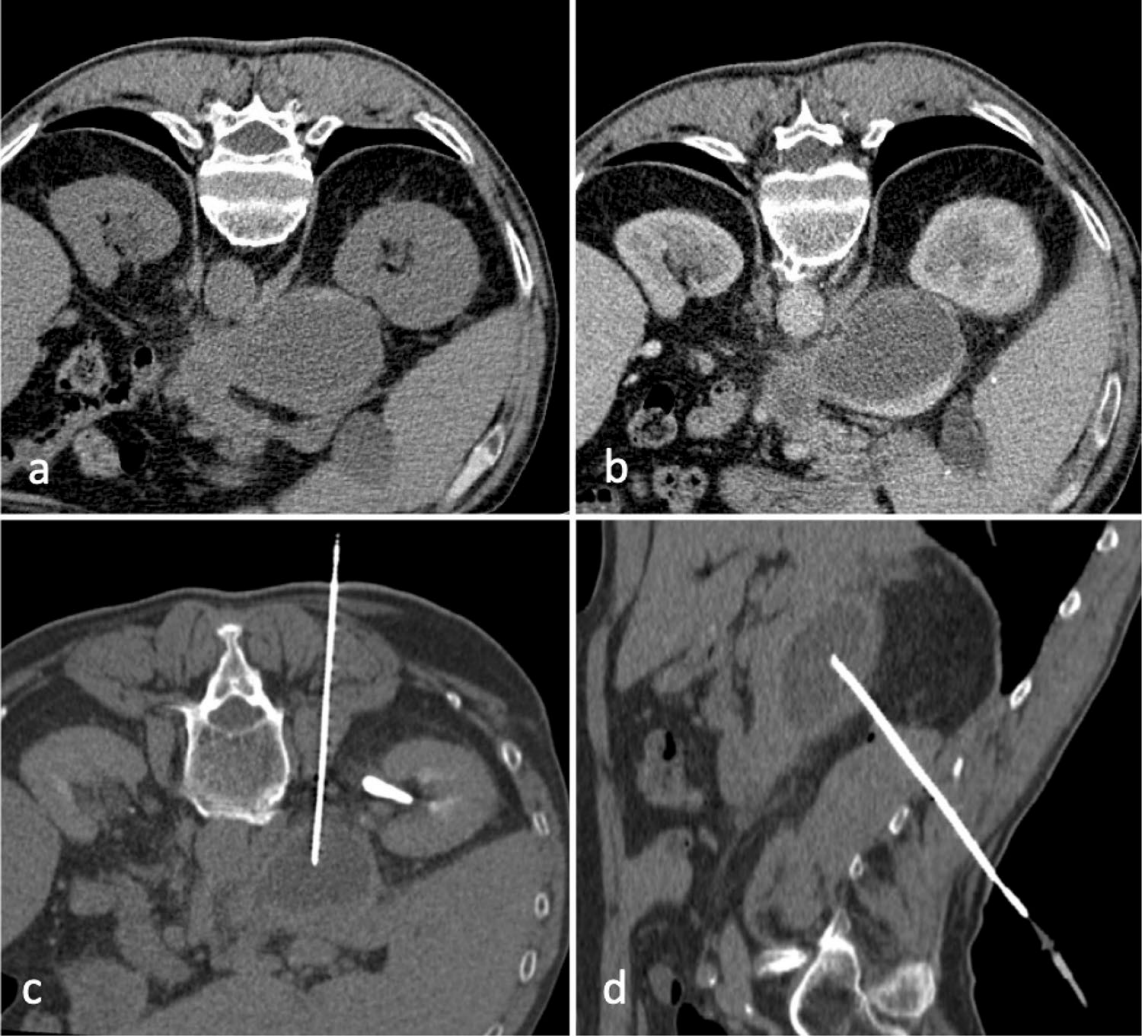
Drainage of a pancreatic pseudocyst in a 65-year-old male patient. Axial pre- and post-contrast CT scans (a, b) point out the interposition of the lung. Using MPR reconstructions, the lesion is reached through a caudocranial path and drained without complications (c, d)
